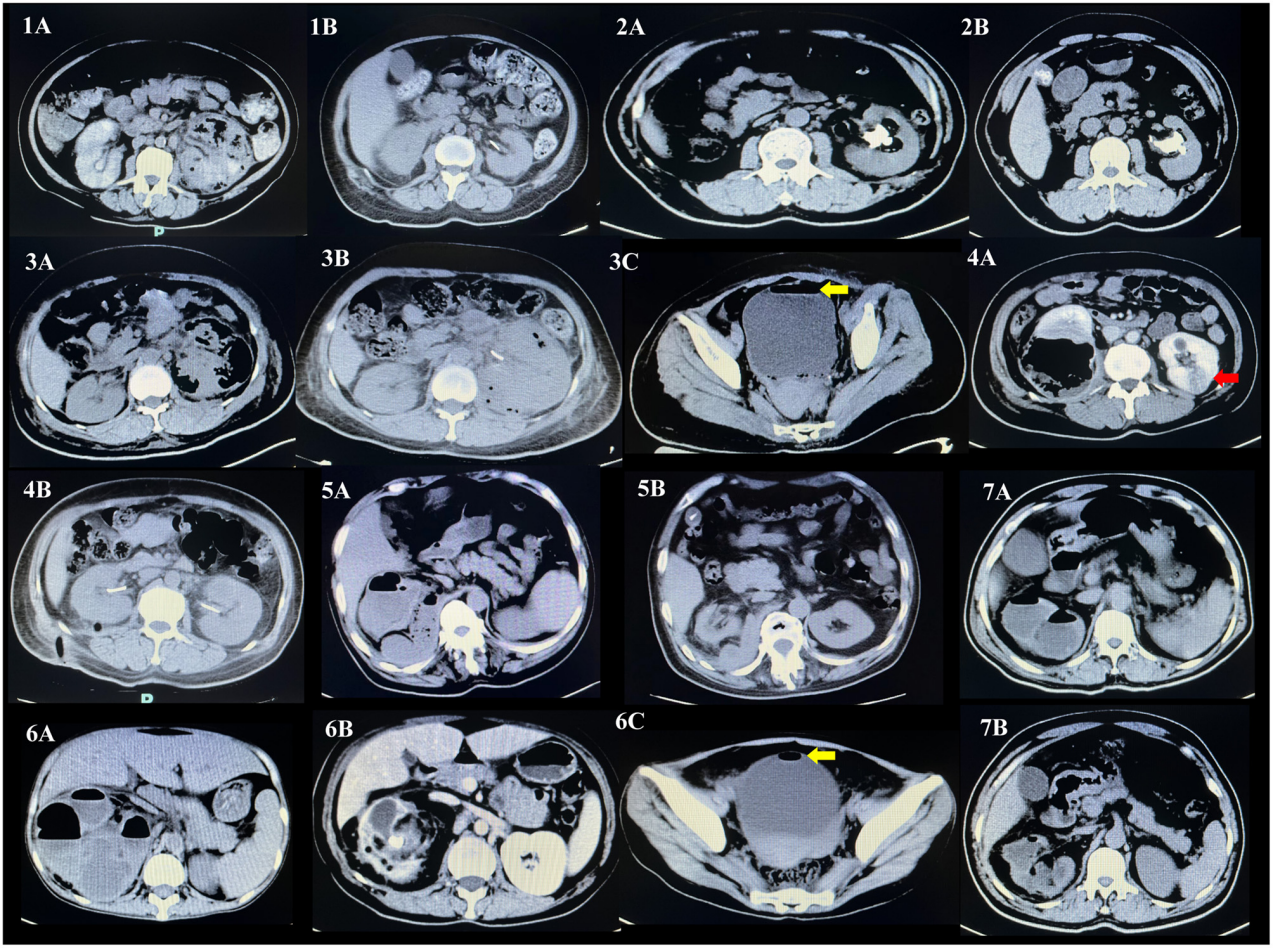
Comparative imaging data before and after operation of patient 1–7. Preoperative CT scan results: (1A−7A). Recheck CT scan results 1 week after operation: (1B−7B). Complicated with emphysematous cystitis: (3C, 6C). The yellow arrow indicates emphysematous cystitis. Complicated with left kidney abscess: (4A). The red arrow points to a renal abscess.
